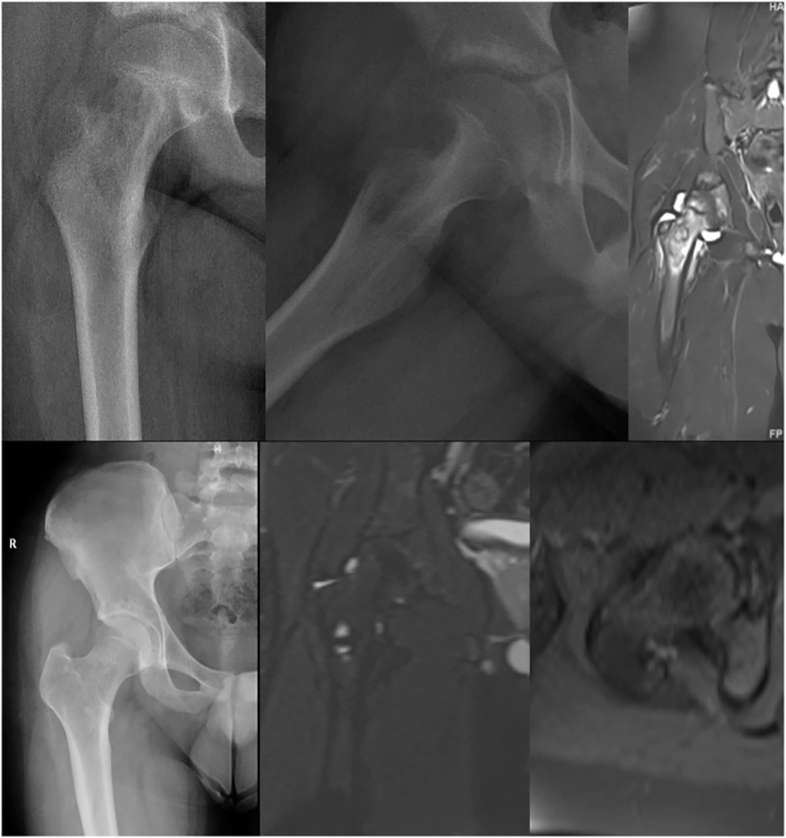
Radiographs and MRI images of a 19-year-old male patient diagnosed with LCH in the right proximal femur before RFA and after a 4-year follow-up.
